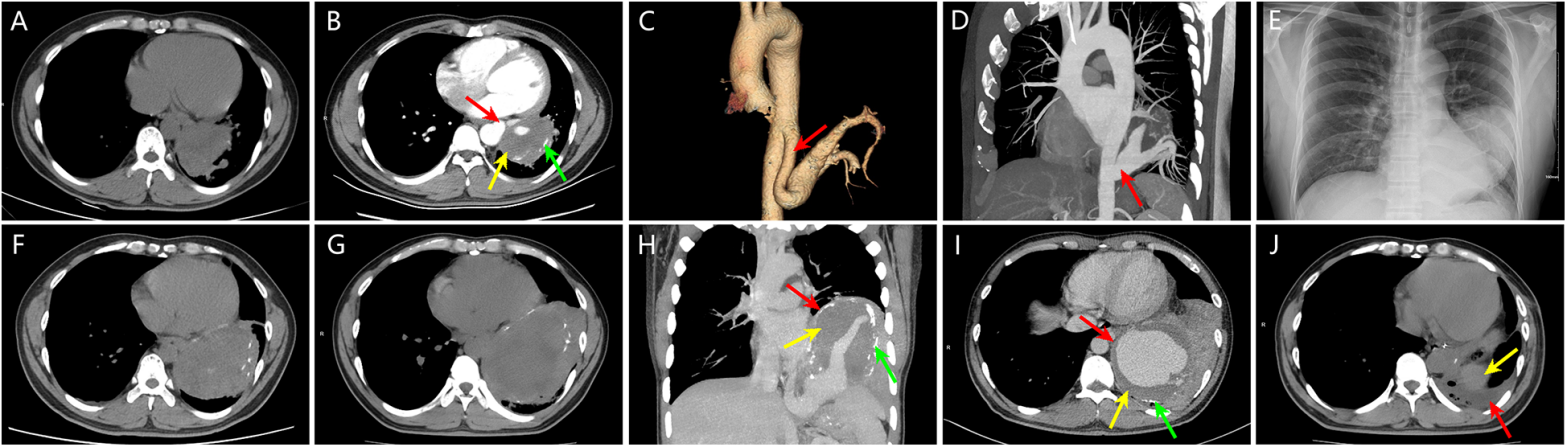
(A) Nine years ago, chest CT revealed a mass in the left lower lobe measuring approximately 74 mm × 61 mm. (B) Nine years ago, contrast-enhanced chest CT showed a clearly defined feeding artery (red arrow) supplying the left lower lobe mass, with a maximum diameter of approximately 65 mm × 43 mm, a normal proximal diameter of about 12 mm, indicating vascular dilation of at least 3.6 times; mural thrombosis within the vascular lumen (yellow arrow) and calcified plaques in the vessel wall (green arrow) were also observed. (C, D) Nine years ago, post-aortic angiography volume rendering (VR) and maximum intensity projection (MIP) images clearly depicted the feeding artery originating from the thoracic aorta (red arrow), confirming the diagnosis of intralobar pulmonary sequestration complicated by a supplying artery aneurysm. (E, F) One year ago, chest x-ray and CT showed a left lower lobe mass measuring approximately 112 mm × 82 mm, indicating significant enlargement compared to nine years earlier. (G) At this visit, chest CT revealed the left lower lobe mass measuring approximately 137 mm × 96 mm, further increasing in size compared to one year ago. (H, I) At this visit, contrast-enhanced chest CT and MIP images displayed a giant aneurysm of the sequestrated lung and its supplying artery (red arrow), with the aneurysm's maximum diameter measuring approximately 123 mm × 100 mm, with a normal proximal diameter of about 13 mm, indicating vascular dilation of at least 7.7 times. Mural thrombosis within the vascular lumen (yellow arrow) and calcified plaques in the vessel wall (green arrow) were also visible. (J) One month post-surgery, follow-up chest CT revealed small pleural effusion (red arrow), with the yellow arrow indicating the spleen.
